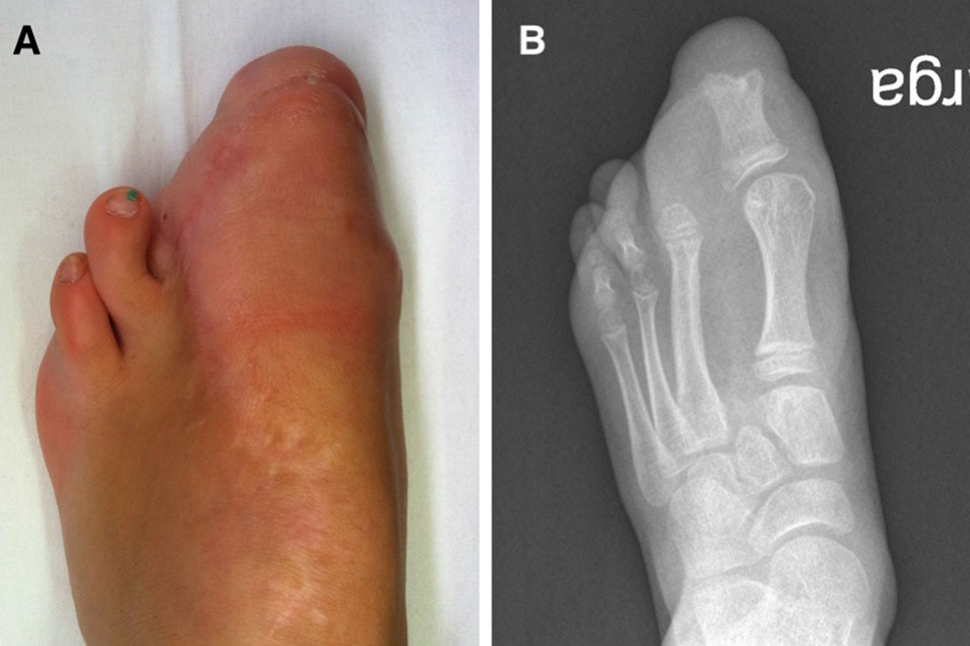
Case 2: Clinical photograph (a) and radiograph (b) at 1-year follow-up
